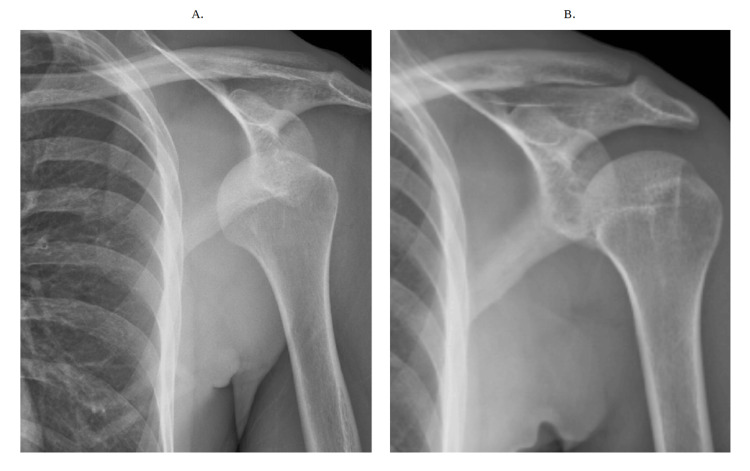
Repeat X-ray of the shoulder shows a dislocation of the left shoulder (a) Anterior shoulder dislocation is present with some re-demonstration of exostosis arising from the coracoid process and well-corticated ossification anterior to the shoulder. (b) Proper interval reduction of anterior shoulder dislocation
